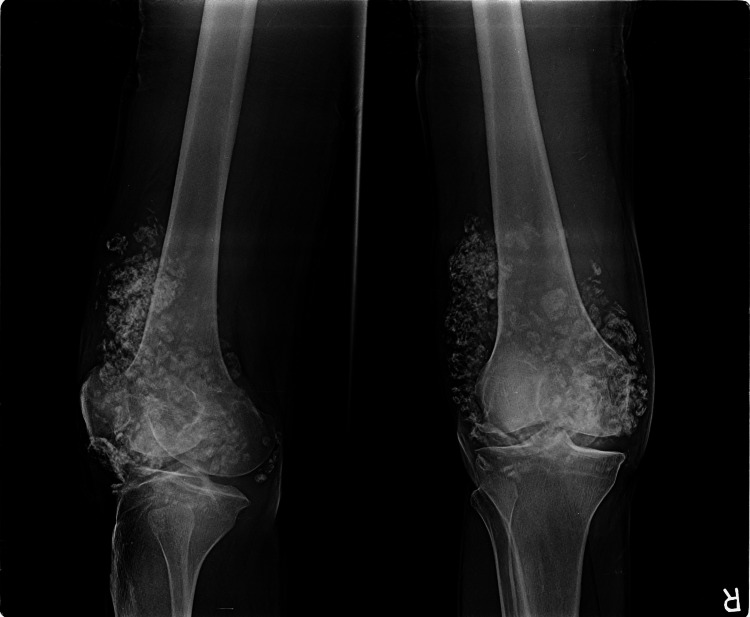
X-ray of the right knee, lateral and AP views. Note the presence of multiple intraarticular calcifications.
